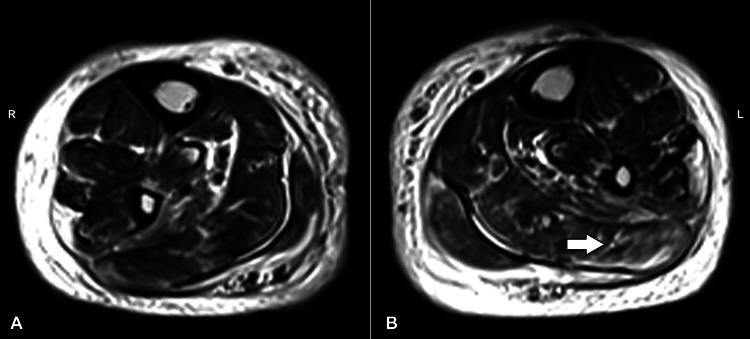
MRI coronal section showing affected areas of the (A) right and (B) left muscles (white arrow) MRI: Magnetic resonance imaging
